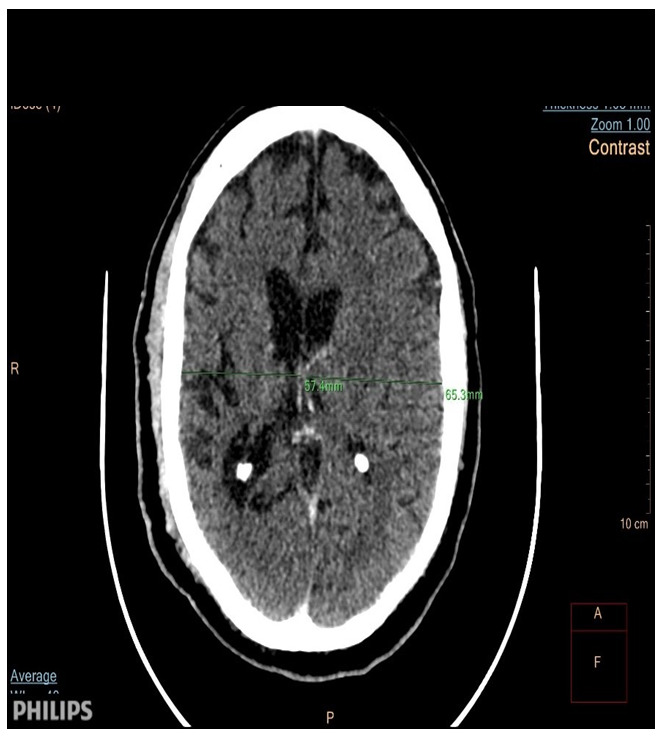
CT scan of the brain (axial view). Shows asymmetry of the lateral ventricles (right to left) with a notable atrophy of the right cerebral hemisphere.
